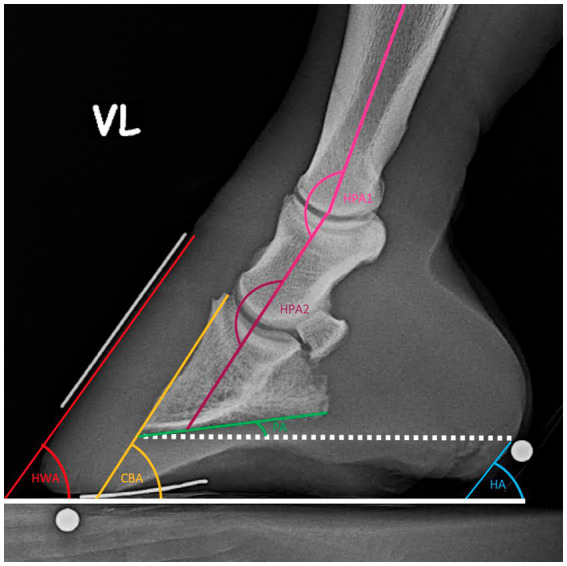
Angular measurements on the lateromedial X-Ray.
